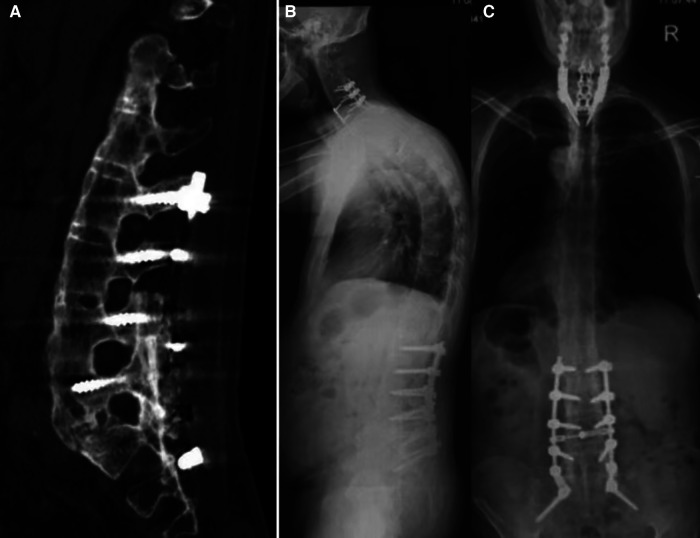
CT (A) and x-rays (B,C) at the most recent follow-up of the spine demonstrating healing of the fracture.
